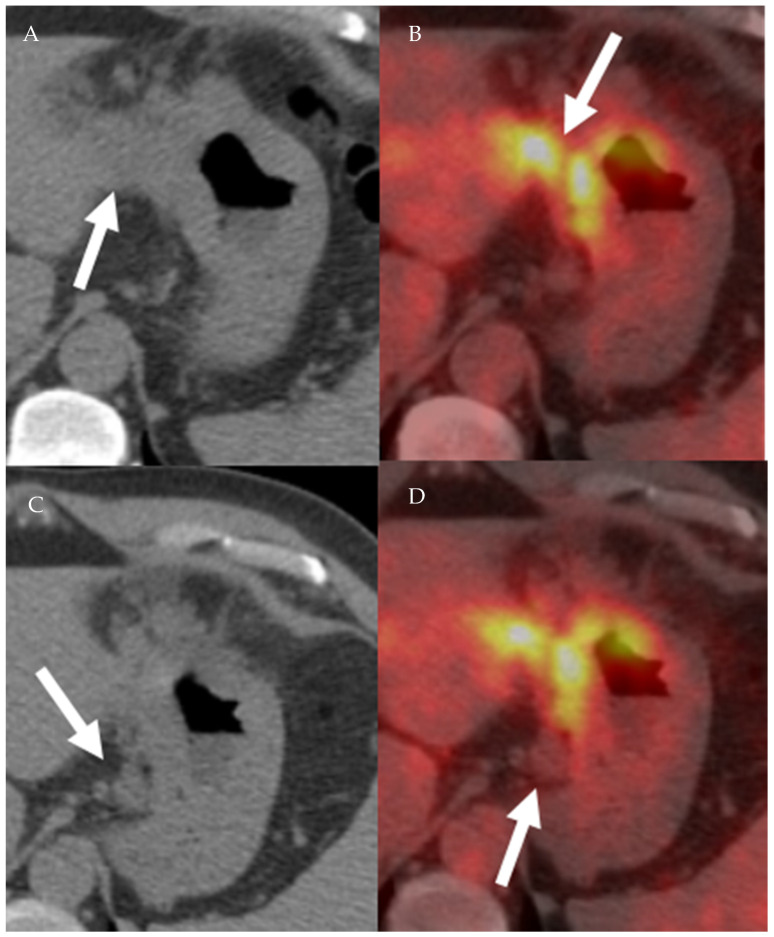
A 77-year-old male patient with gastric cancer. PET/CT scan demonstrates marked thickening of the gastric antrum with increased uptake (A,B) consistent with gastric carcinoma, maximum SUV = 8.7 (B). Enlarging metastatic adenopathy in the gastrohepatic ligament is also noted (C,D), not hypermetabolic on PET/CT.
